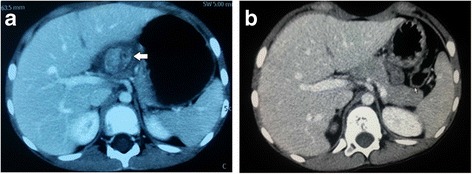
a Contrast-enhanced abdominal computed tomography showed there was a mass in the hepatic portal area (*left arrow*), the size of which was approximately 3.2 × 4.3 cm, and it slightly adhered to the surrounding tissue. The mass was not uniformly enhanced, the center of the tumor had obvious enhancement, and there was no significant invasion of our patient’s liver. b Contrast-enhanced abdominal computed tomography after operation
